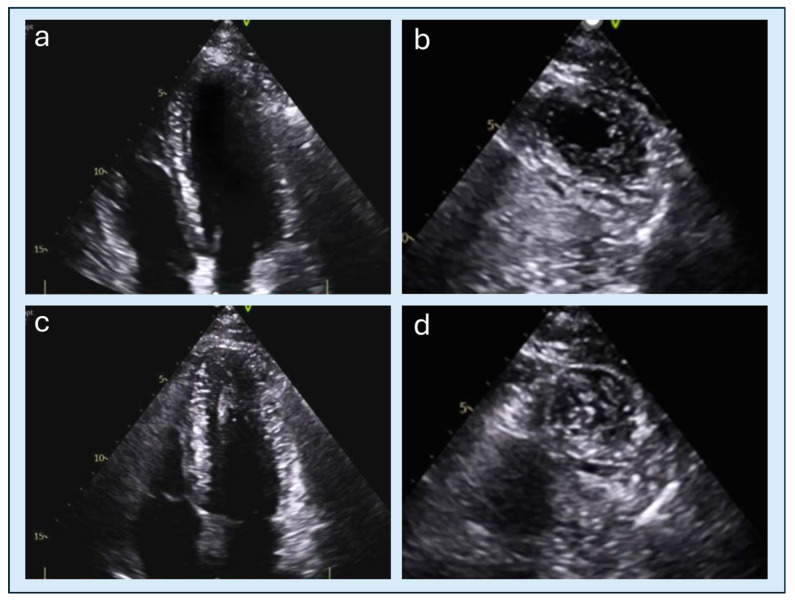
TTE assessment of apical obliteration. (a) apical four-chamber long-axis view in diastole, showing a preserved apical cavity. (b) focused short-axis view in diastole, confirming a residual apical lumen. (c) apical four-chamber long-axis view in systole, demonstrating near-complete obliteration of the apical cavity. (d) focused short-axis section in systole, showing circular endocardial apposition consistent with apical obliteration.
